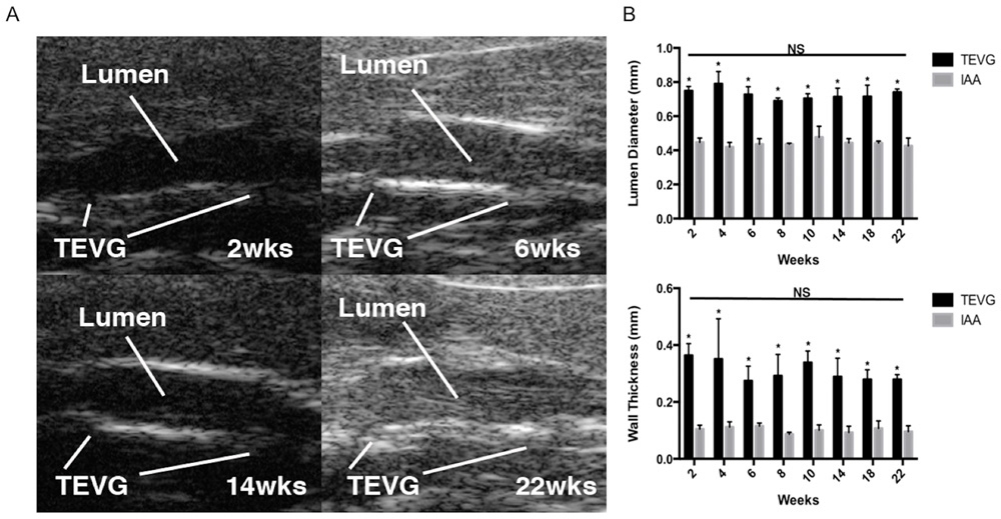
Preliminary study results. Representative Doppler ultrasound images of TEVGs at various time points showing graft patency *in vivo* (A). TEVG lumen diameter and wall thickness as measured by Doppler ultrasound (n = 3). Data in graphs are expressed as mean ± SD. P* <0.05. There was a statistically significant difference in both luminal diameter and wall thickness between TEVG and IAA at each time point. However, no statistically significant difference (NS) was found between all time points for either TEVG or native IAA (B).
